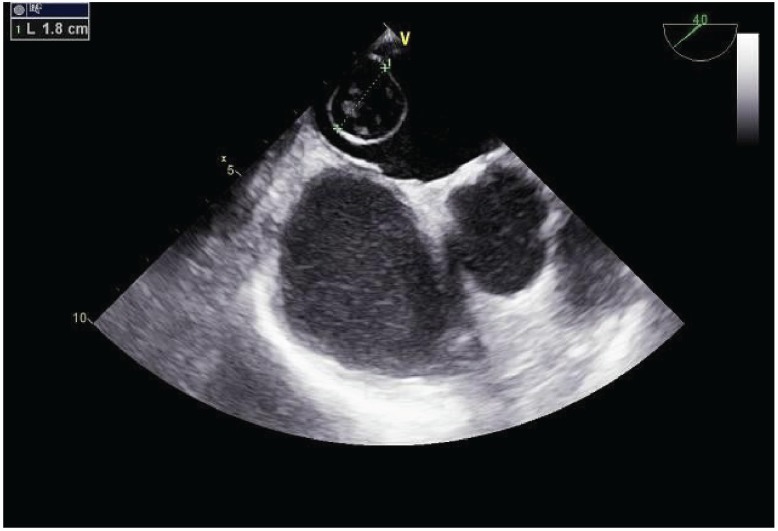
Transesophageal echocardiogram of the mass in the left atrium.
